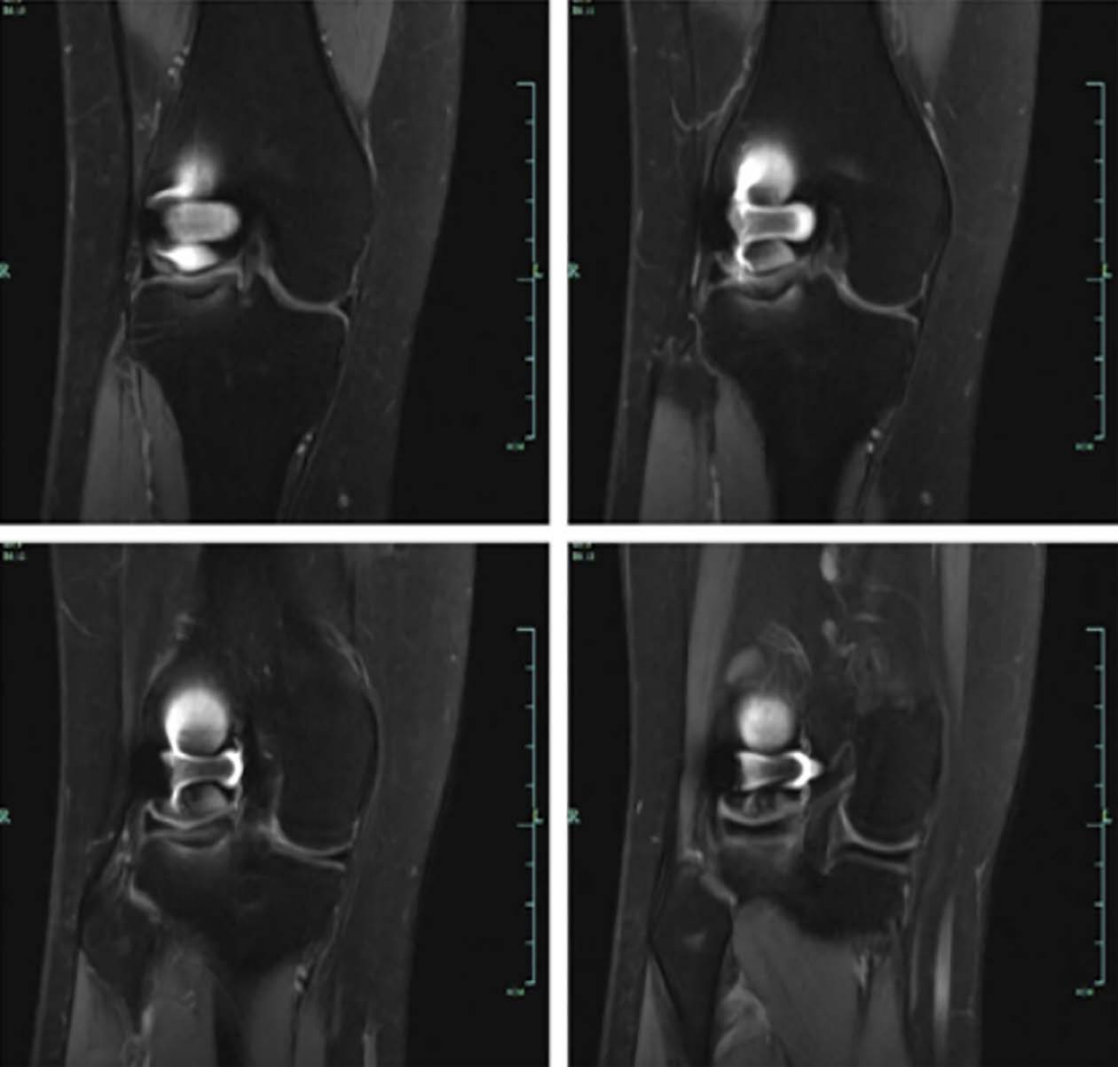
Coronal MRI images were reexamined 18 months after operation, MRI = magnetic resonance.
